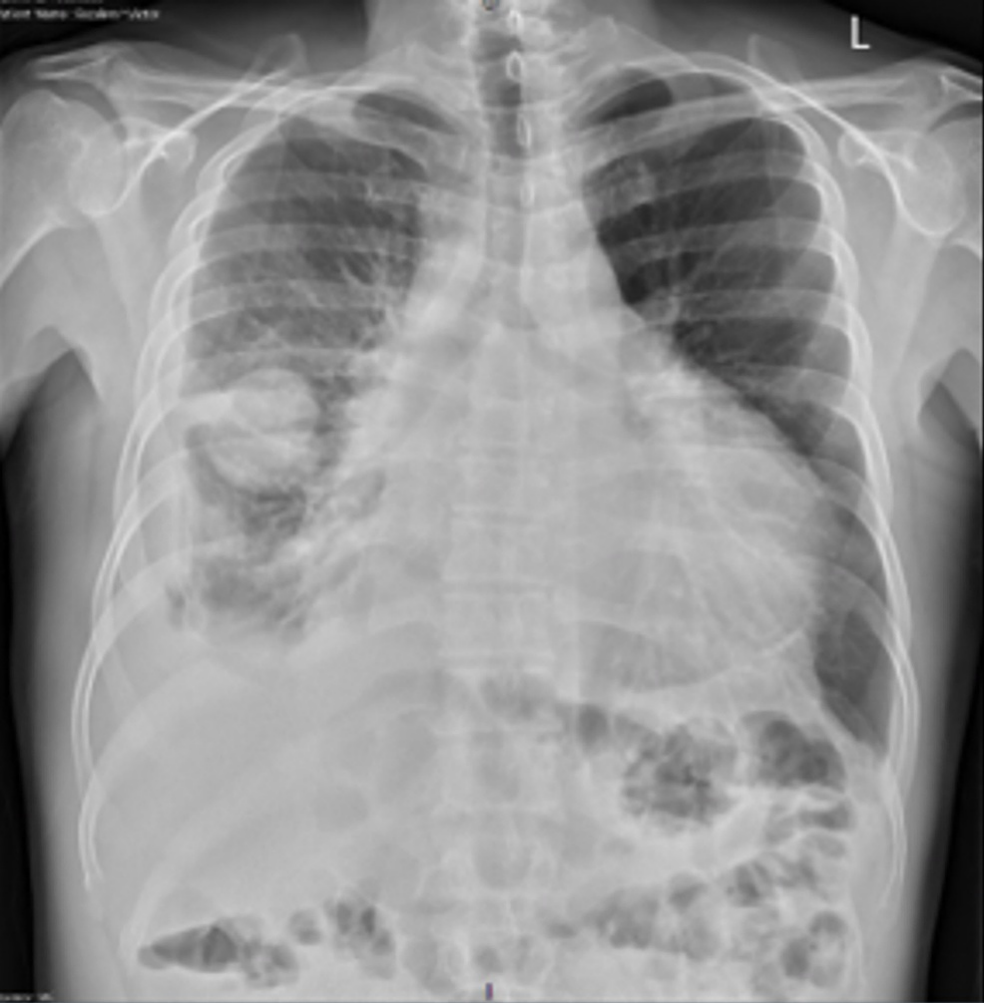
Chest X-ray of the patient The image shows mass projecting over the anterior mid lung in the region of the minor fissure, enlarged cardiac silhouette, and trace left pleural effusions
